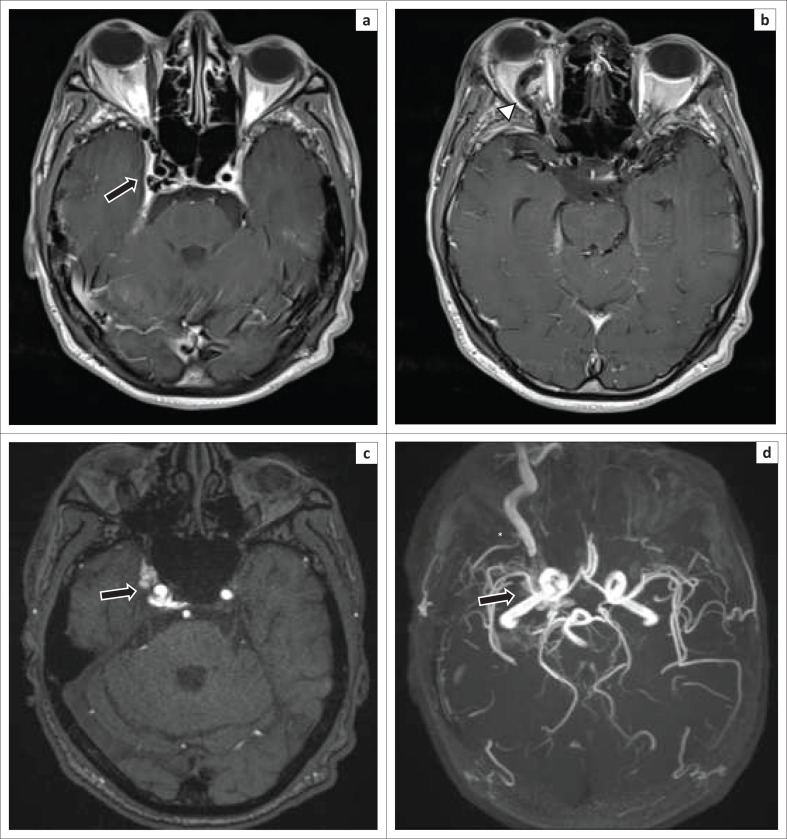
A 39-year-old male presented with proptosis and chemosis 6 months after being involved in a motor vehicle accident: (a) Axial T2W magnetic resonance imaging shows abnormal flow voids within the right cavernous sinus (arrow) as well as proptosis of the right globe. (b) Additional T2W slices in the same patient demonstrate a dilated ipsilateral superior ophthalmic vein (arrowhead). (c) Flow-related hyperintensity is noted on the MR time-of-flight (TOF) source images. (d) The corresponding MR TOF 3D MIP images display a dilated right superior ophthalmic vein (asterisk) with enhancement in the region of the right cavernous sinus (arrow).
